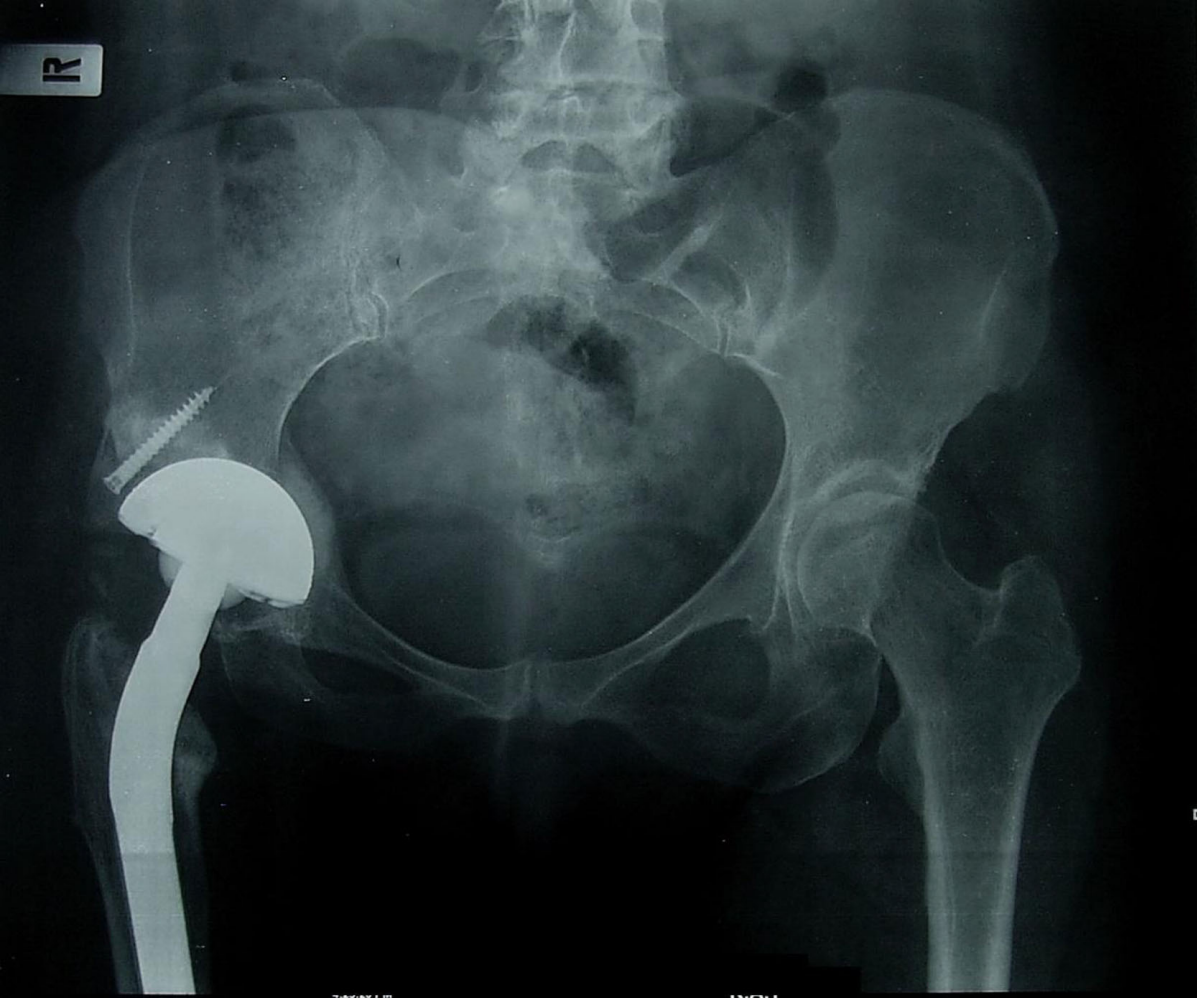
Pelvis anteroposterior X-ray at 3 months postoperatively. The cup has no sign of migration. The satisfactory healing process of the morselised graft is seen at the acetabular bed. The structural autograft remains in its initial place held with one screw.
